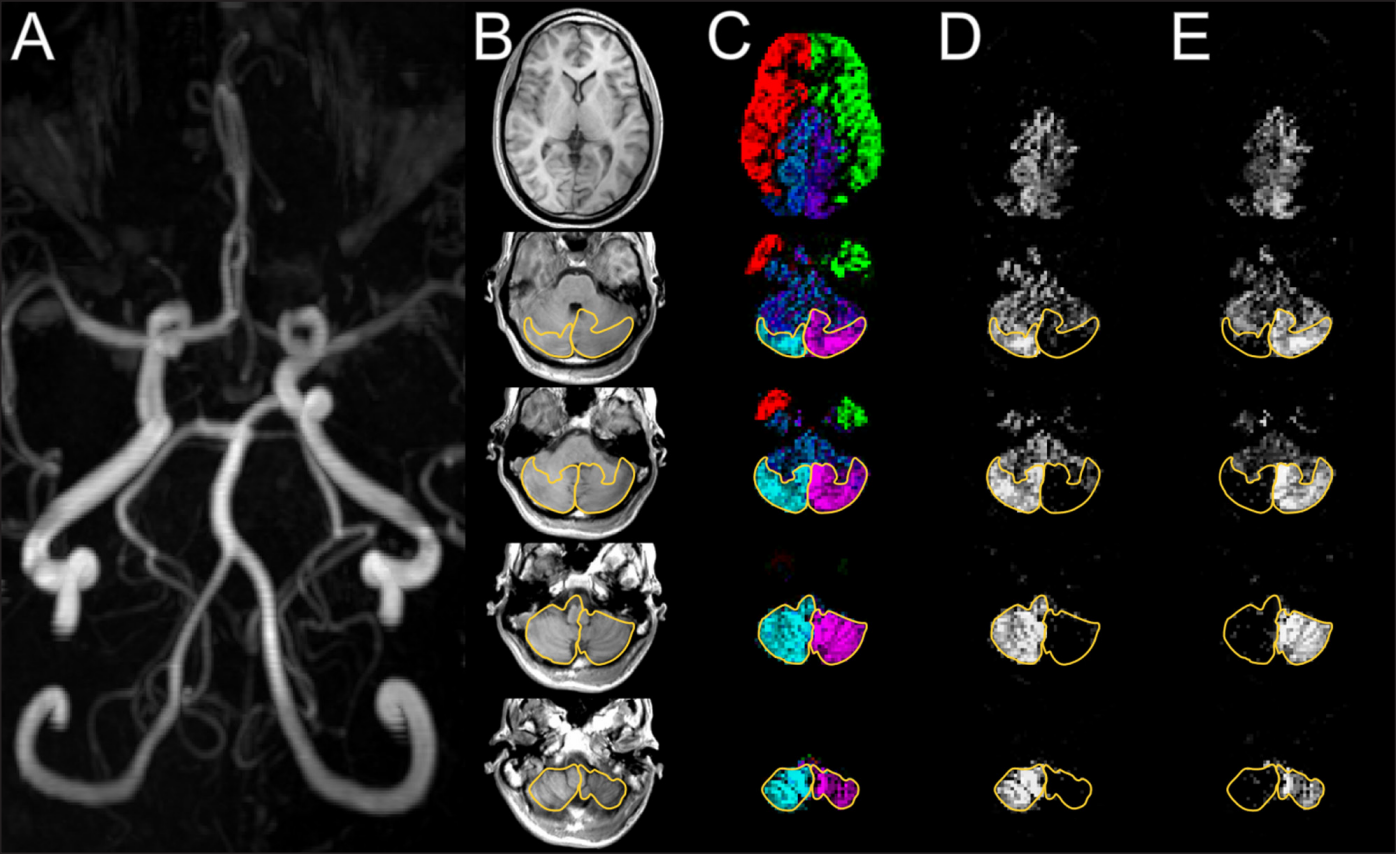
Time-of-flight MRA (A) shows a slightly dominant left vertebral artery. The anatomical T1-weighted images (B) show through the cerebellum from inferior (bottom row) to superior (top row). Territorial perfusion images (C) show the territory supplied by the right (cyan) and left (magenta) vertebral arteries and by the right (red) and left (green) internal carotid arteries. With the mixing of blood in the basilar artery, cyan and magenta turn into blue. Individual perfusion images of the right (D) and left (E) vertebral arteries. The posterior inferior cerebellar artery (PICA) territories are outlined in perfusion images (C–E) and copied to the anatomical images (B). The PICA territories can be easily discriminated; they are symmetrical and supply the posterior cerebellar surfaces (B). Reproduced from *Neuroimage* [2].
